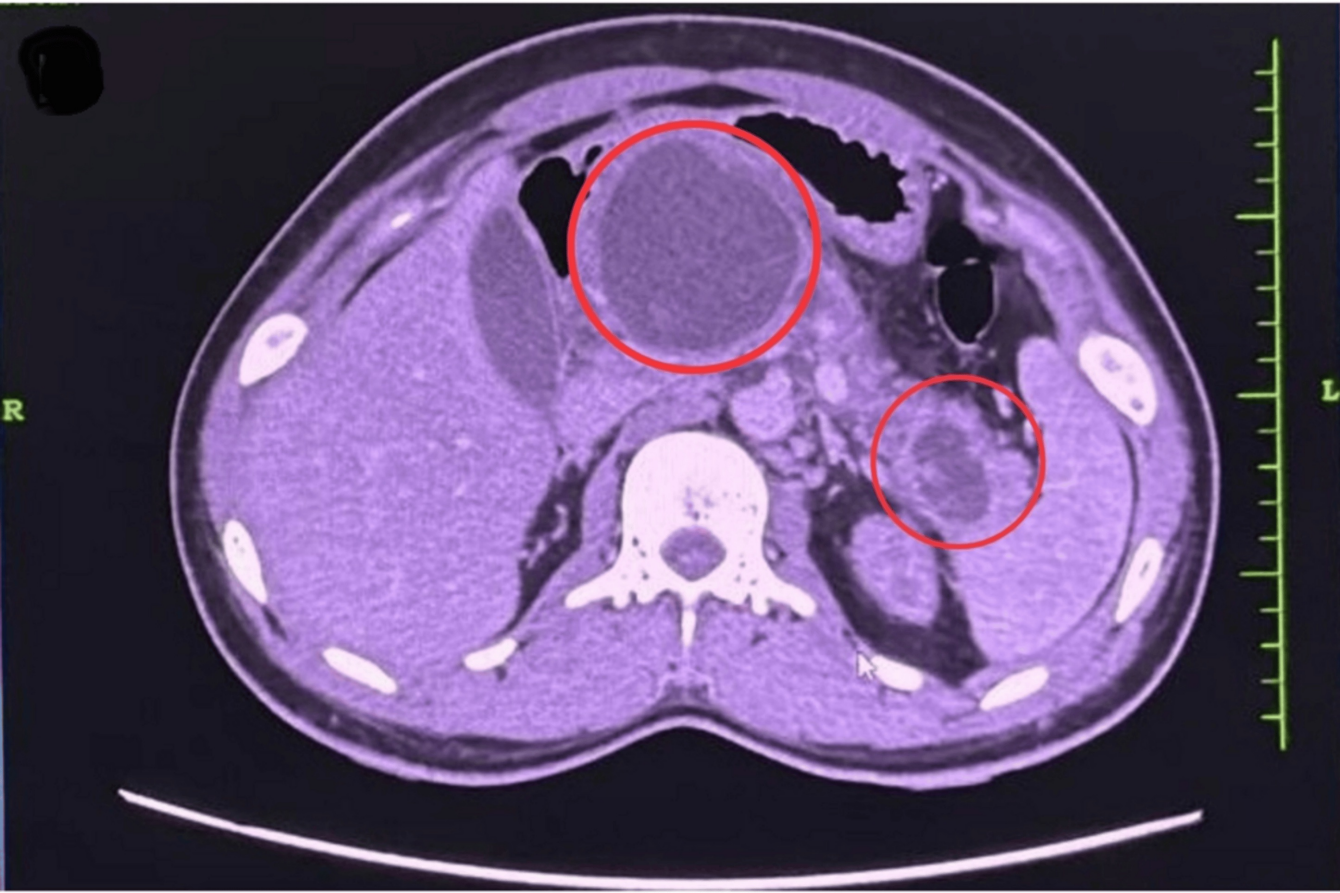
CT abdomen showing a well-defined, encapsulated fluid collection with heterogeneous internal contents, consistent with walled-off pancreatic necrosis (WOPN).
